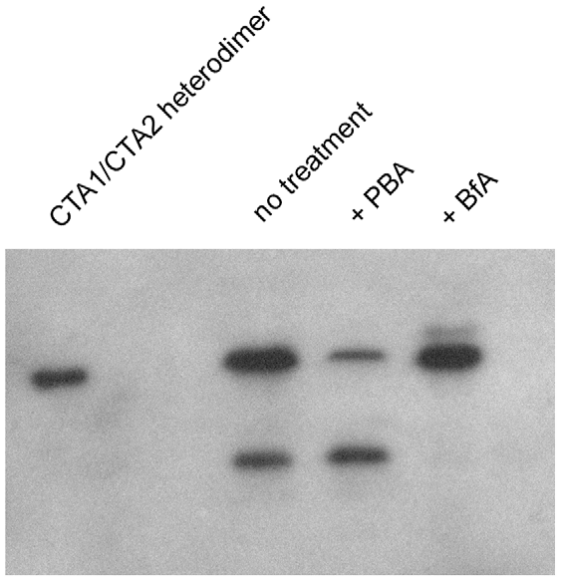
PBA does not block reduction of the CTA1/CTA2 disulfide bond in the ER. HeLa cells pulse-labeled at 4°C with recombinant CT were chased for 2 hr at 37°C in medium containing no additions (no treatment), 100 µM PBA, or 5 µg/ml of BfA. Membrane fractions from digitonin-permeabilized cells were resolved by non-reducing SDS-PAGE and probed by Western blot with an anti-CTA antibody. A purified, disulfide-linked CTA1/CTA2 heterodimer was also run as a control. We consistently detected slightly more reduced CTA1 in PBA-treated cells than in the untreated control cells (*n* = 4).
